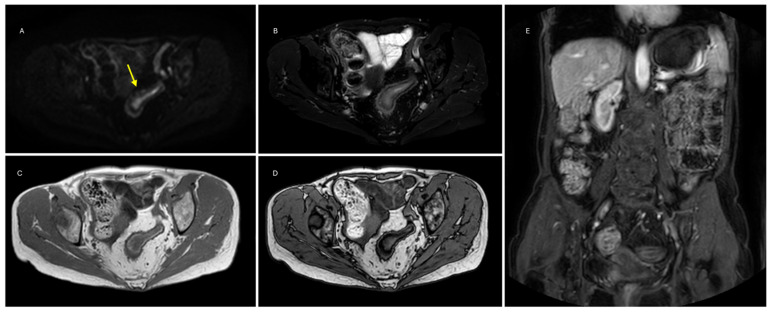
(A) DWI on high b-value image showing the bright luminal sign at the sigmoid tract (yellow arrow). (B) T2w fat-saturation image shows no signs of submucosal edema. (C,D) T1 IP-OOP showing diffuse submucosal adipose infiltrate. (E) Post-contrast image with layered pattern of enhancement at 70 s.
